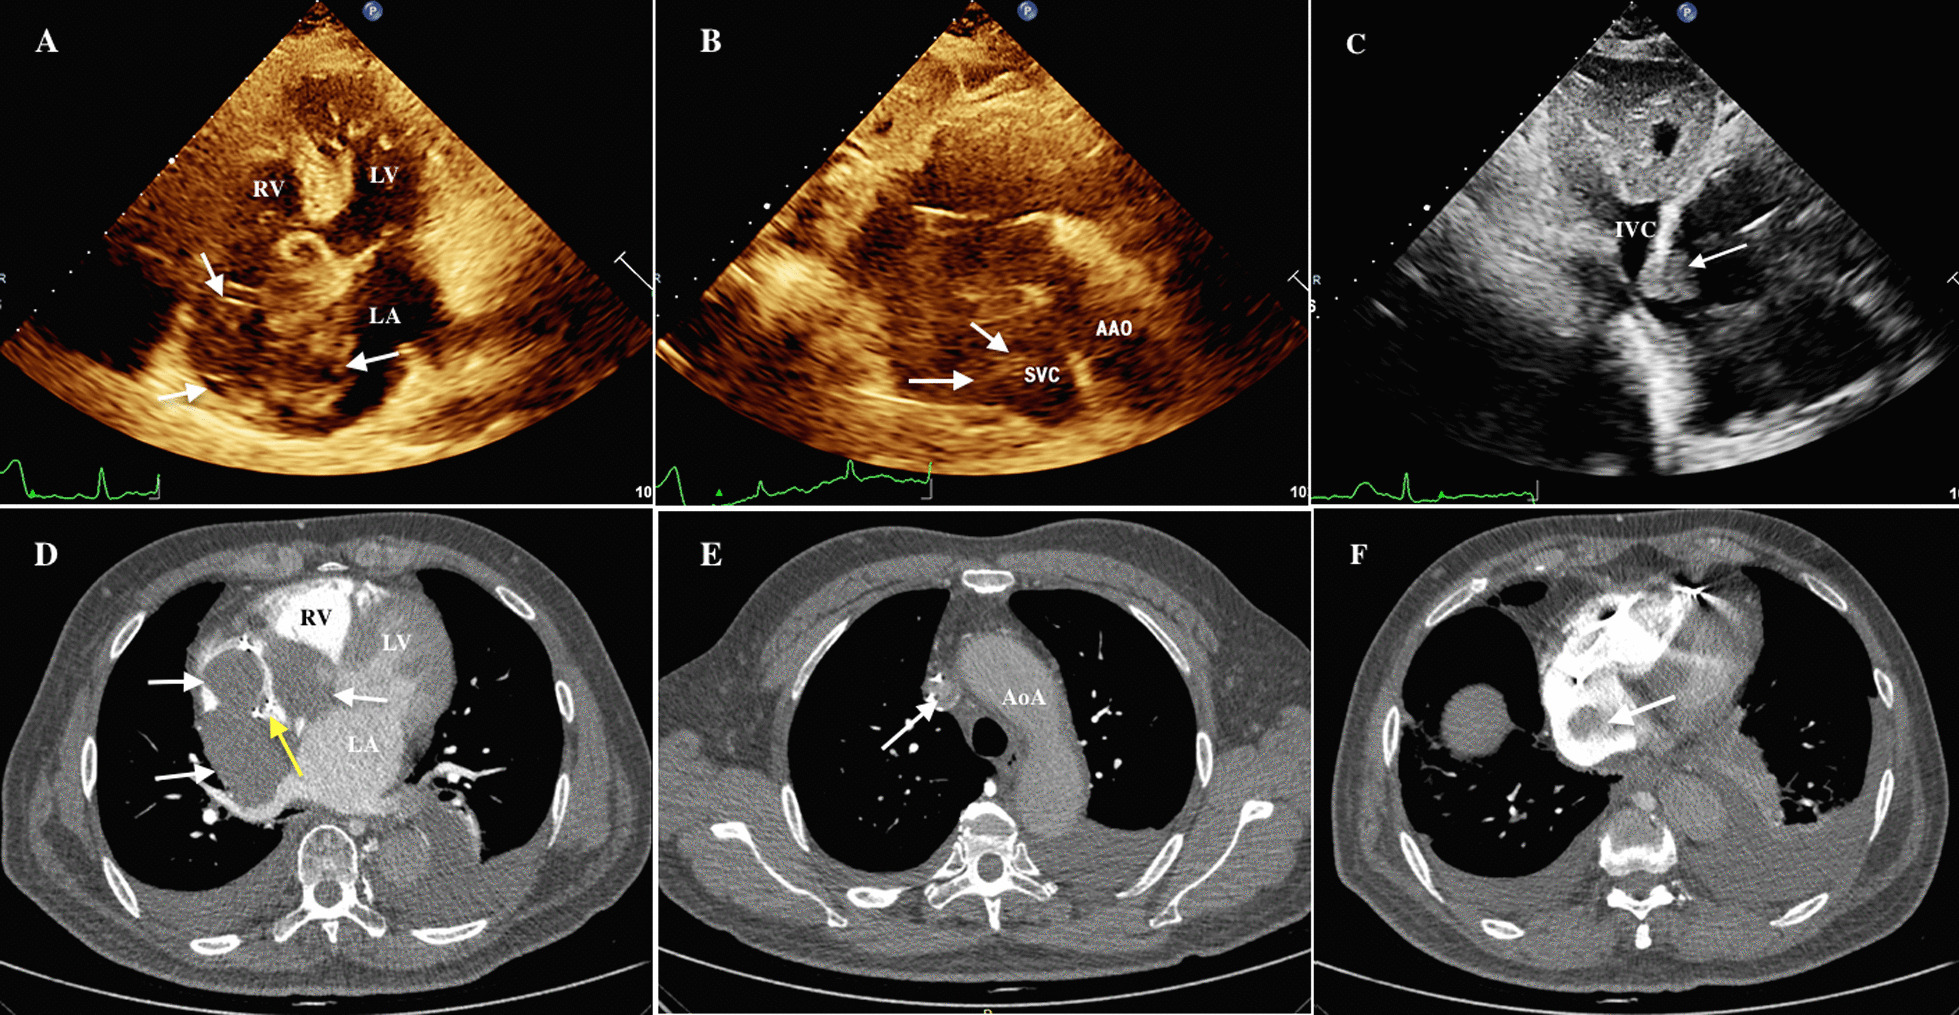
Echo view: a The arrows indicate that the large mass occupied most of the RA. b The arrows indicate that the mass extended into the IVC. c The arrow indicates that the mass extended into the SVC. Transverse view of CTA: d, e and f The arrows demonstrate the large mass in the RA extending into the IVC and SVC. The yellow arrow indicates the mass surrounding the pacemaker lead (d). RA, right atrium; RV, right ventricle; LA, left atrium; LV, left ventricle; AAO, ascending aorta; SVC, superior vena cava; IVC, inferior vena cava; AoA, aortic arch; CTA, computed tomographic angiography
